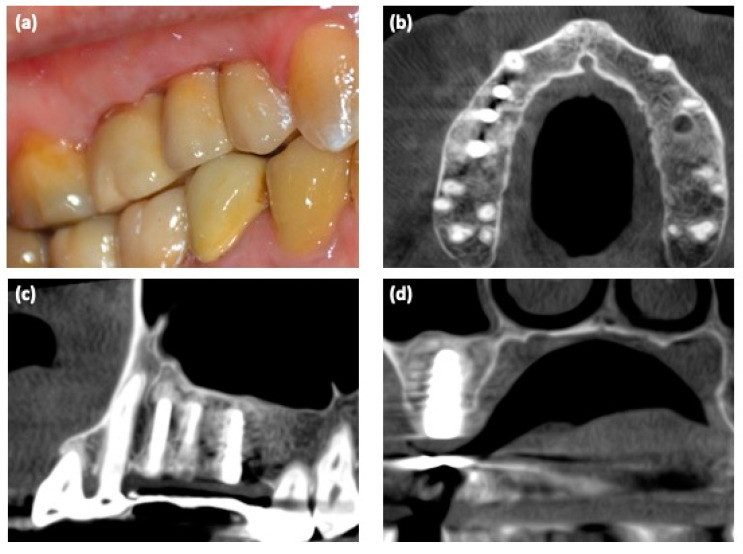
(a) Clinical view of the implant-based rehabilitation; (b–d) 3D CBCT scan after implant placements.
